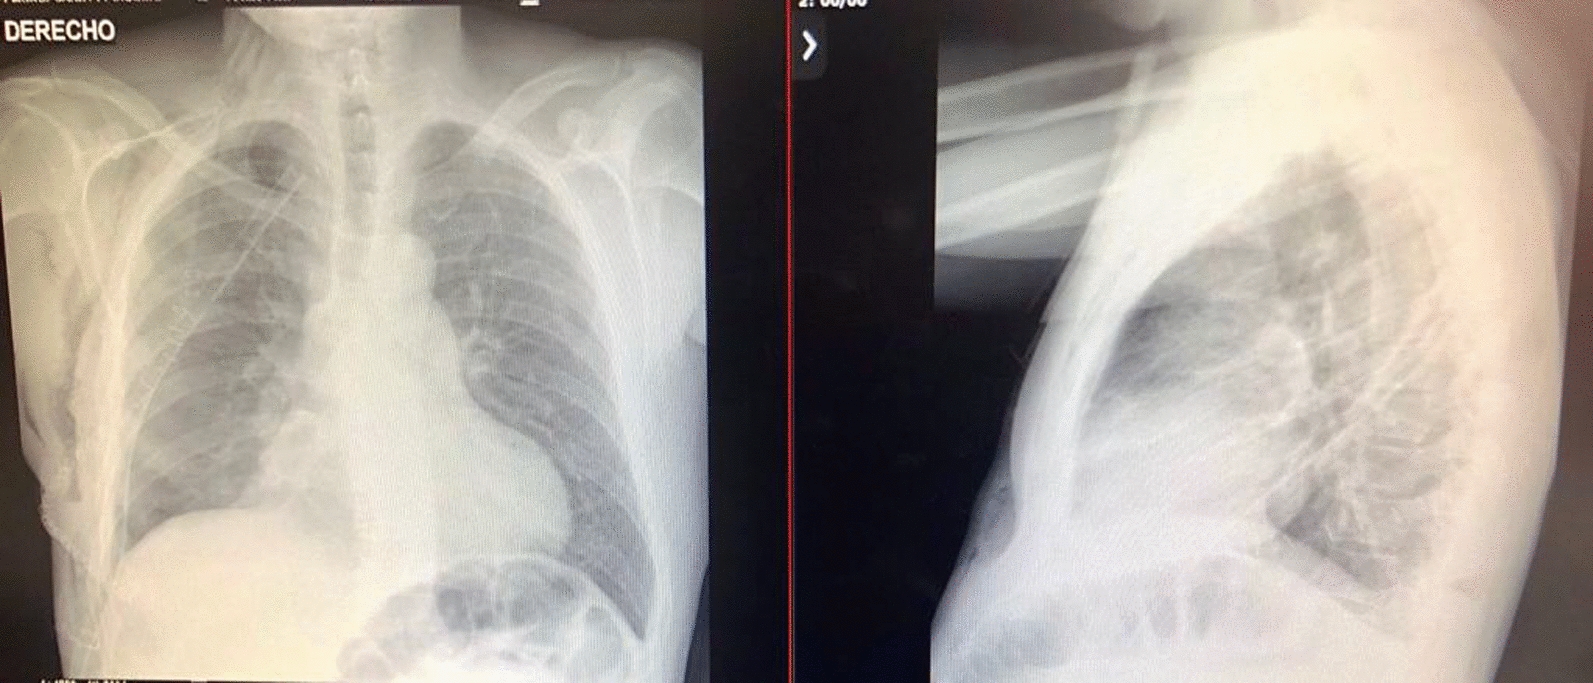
Postoperative anteroposterior (AP) and lateral chest radiography demonstrating lung re-expansion, with clear costophrenic and cardiophrenic angles
